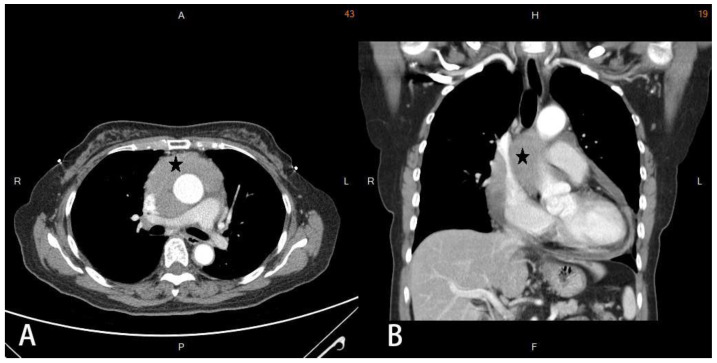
Chest computed tomography with contrast. Axial view (A) and coronal view (B) of chest computed tomography showing confluent soft tissue masses (asterisk) in the intrapericardial space, with an encasement of the aorta and superior vena cava as well as compression of the right pulmonary artery.
